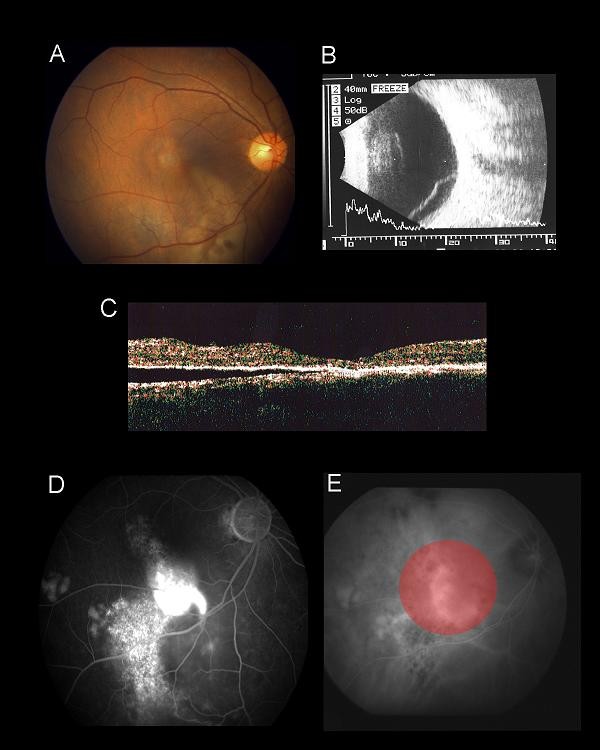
(A) Fundus photo of his right eye at presentation showing exudative retinal detachment at the macula and inferior retina. Fibrinous exudate can be seen at the inferior arcade. (B) B-scan ultrasound axial scan showing inferior exudative retinal detachment. (C) OCT imaging of the right macula showing the presence of sub-retinal fluid involving the fovea. (D) Mid-phase FA showing diffuse fluorescein leakage at the macula with RPE track inferiorly. (E) Mid-phase ICGA showing dilated choroidal vasculature with hyperdynamic circulation consistent with central serous chorioretinopathy. Laser for PDT was applied to the area of choroidal hyperpermeability as guided by ICGA (red circle).
